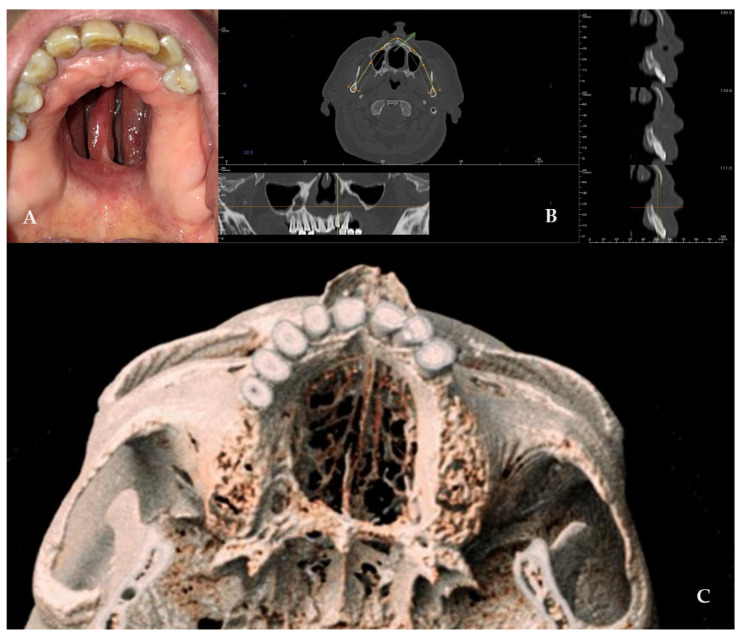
(A) Clinical presentation of the oro-nasal communication. In inset A, it is possible to appreciate the visibility of the nasal septum. (B,C) Radiographic and 3D reconstruction using InVivo6^®^ and Anatomage Table EDU vers.8^®^ (Santa Clara, CA, USA) software. DICOM files were imported in InVivo6^®^ (Santa Clara, CA, USA) software to obtain the axial, cross, and panorex sections. Afterwards, the DICOM files were imported into Anatomage Table EDU vers.8^®^ (Santa Clara, CA, USA) to obtain the 3D rendering.
